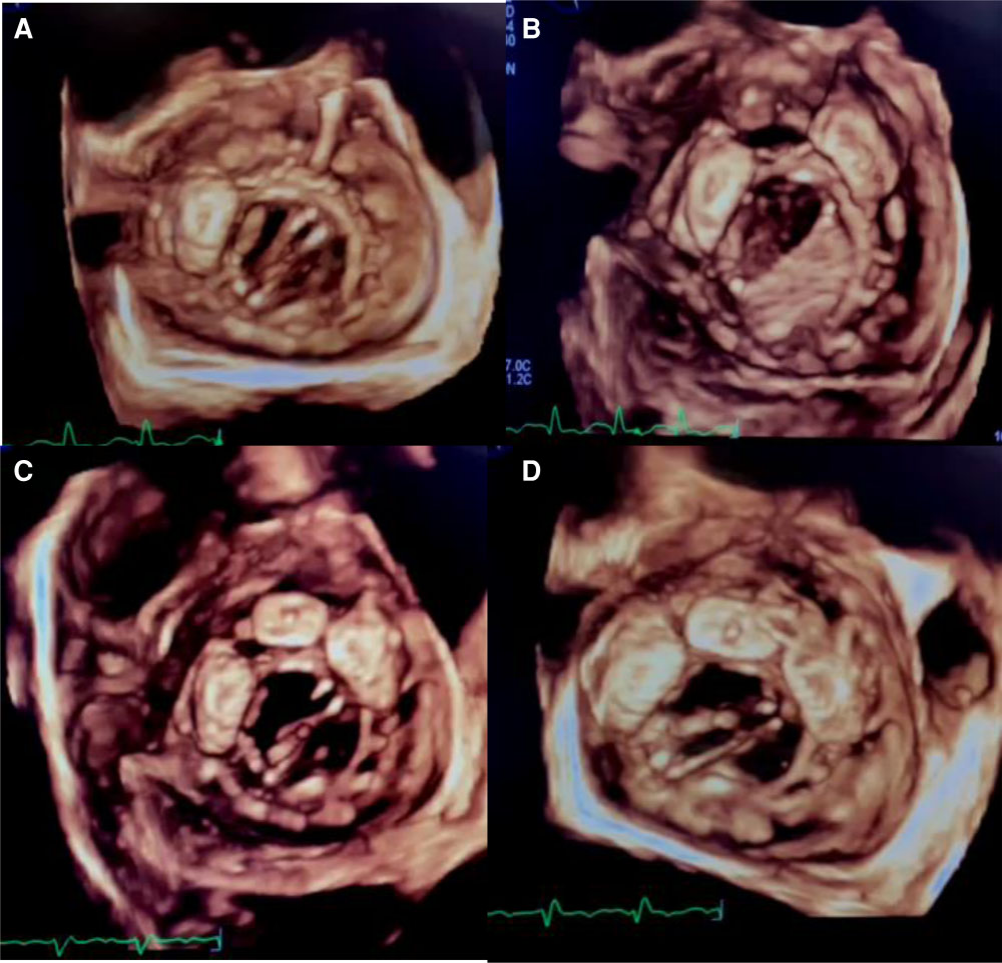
Step-by-step the deployment of closure Device 1 (*A*), Device 2 (*B*), Device 3 (*C*), and a duct occluder (*D*). Supplementary material online, *Video S1*. Transoesophageal echocardiography showing the dehiscence of the prosthetic valve and the paravalvular regurgitation with colour Doppler. Supplementary material online, *Video S2*. Intraprocedural transoesophageal echocardiography showing the closure devices and colour Doppler imaging at the end of procedure.
